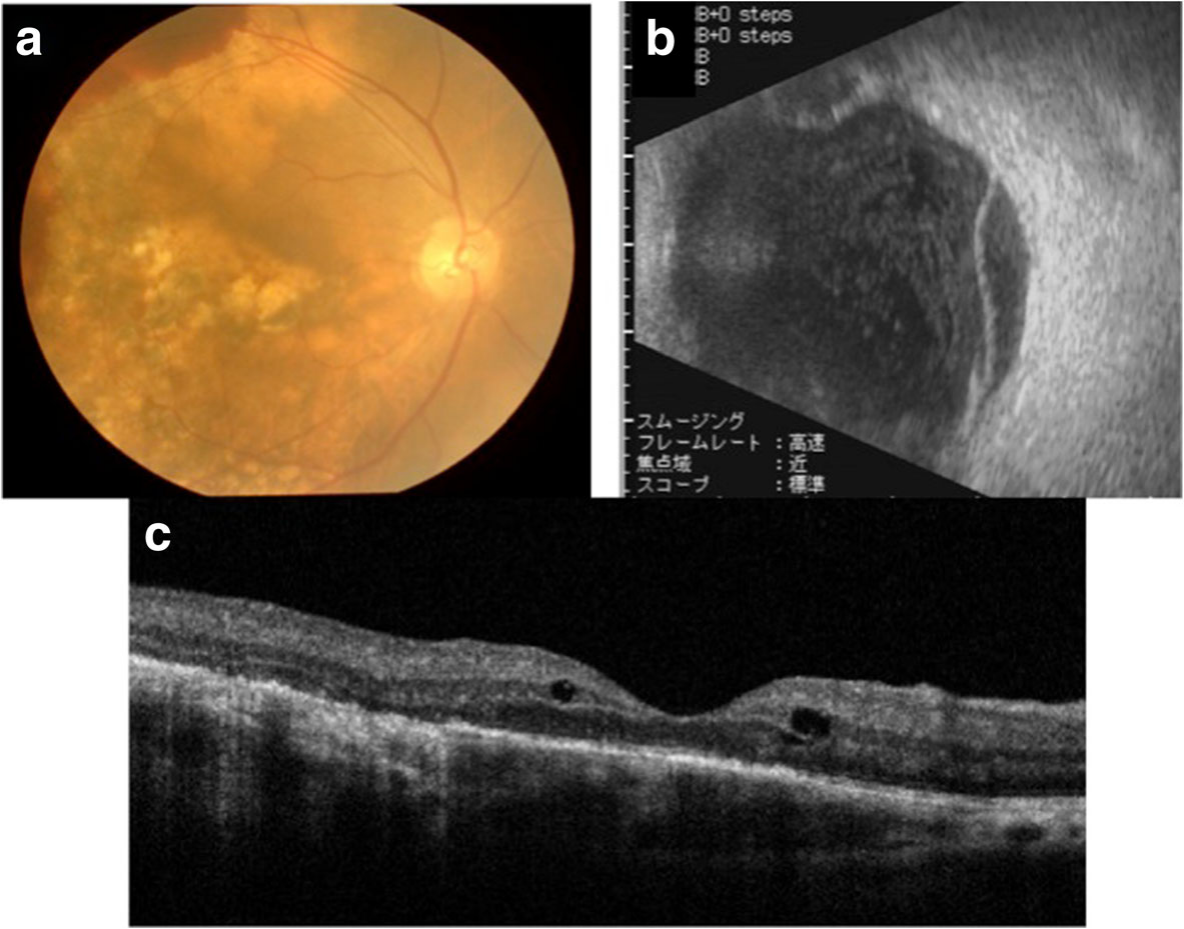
Ophthalmologic examinations. a Fundoscopic examination. b Ultrasonography. c Optical coherence tomographic examination
